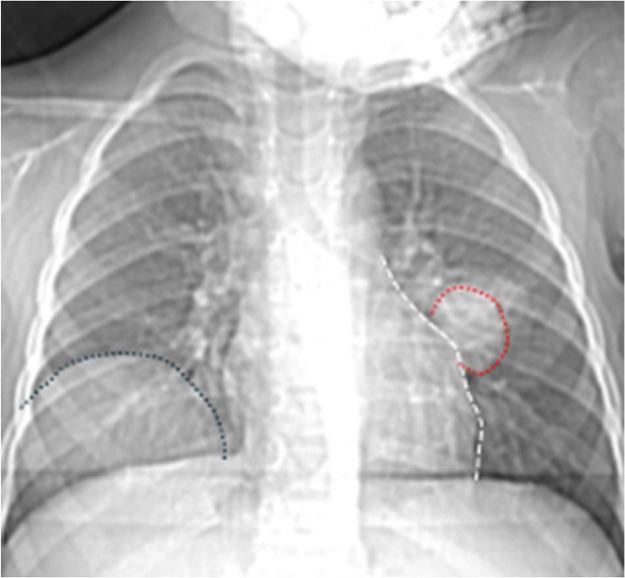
Anteroposterior chest radiograph reveals 2 well-circumscribed, rounded opacities: one located in the right lower lobe (black dotted line) and the other in the left lower lobe (red dotted line). The cardiac silhouette remains clearly demarcated (white dotted line), indicating a negative silhouette sign.
